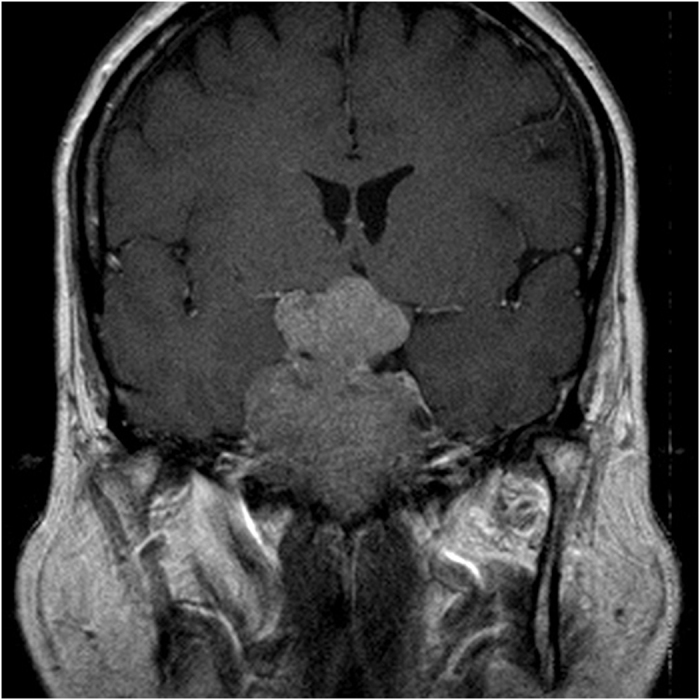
Preoperative MRI of a 64-year-old female patient revealed an extremely large tumor.
